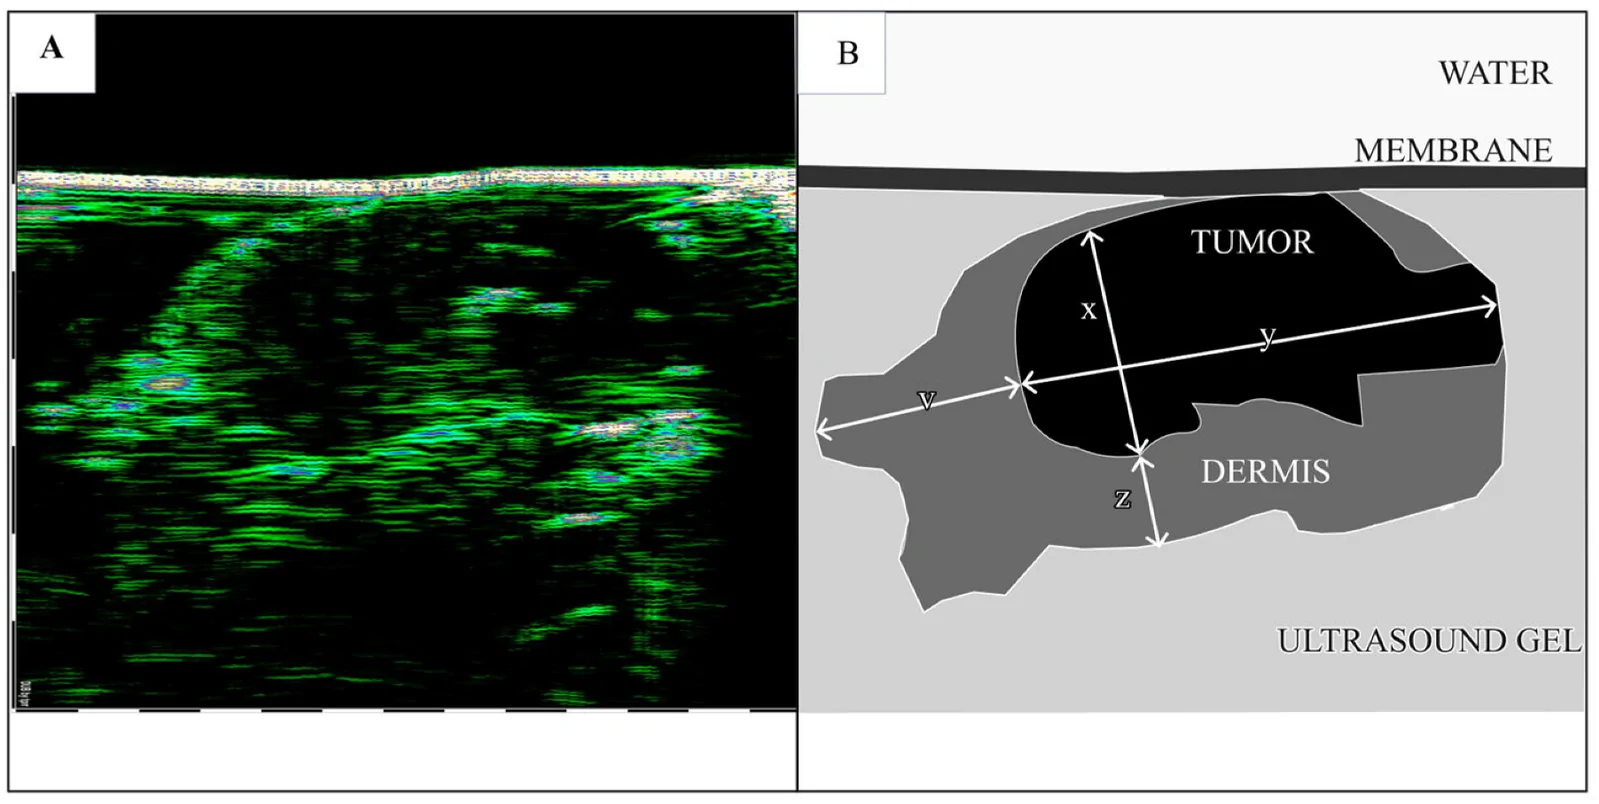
(A) The image obtained from a 22 MHz ultrasound of a skin biopsy. Note the black and white lines on the edge of the image representing millimeters. (B) The main elements of the ultrasonic image are summarized: x—depth of invasion—the depth of the tumor; y—tumor size (greatest dimension); z—the distance between the tumor and the edge of the sample, also named deep margin or tumor free deep limit of excision; v—the distance between the tumor and the lateral border of the skin sample, also named lateral margin.
